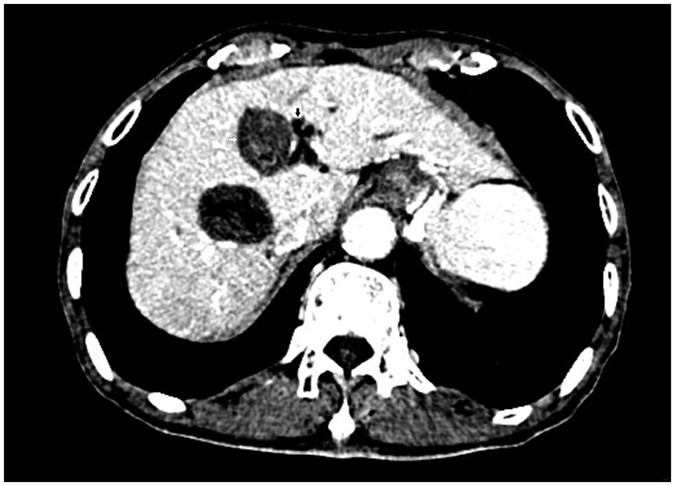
Lesion (white arrow) pushing the left bile duct (black arrow) after ablation as shown with enhanced CT.
